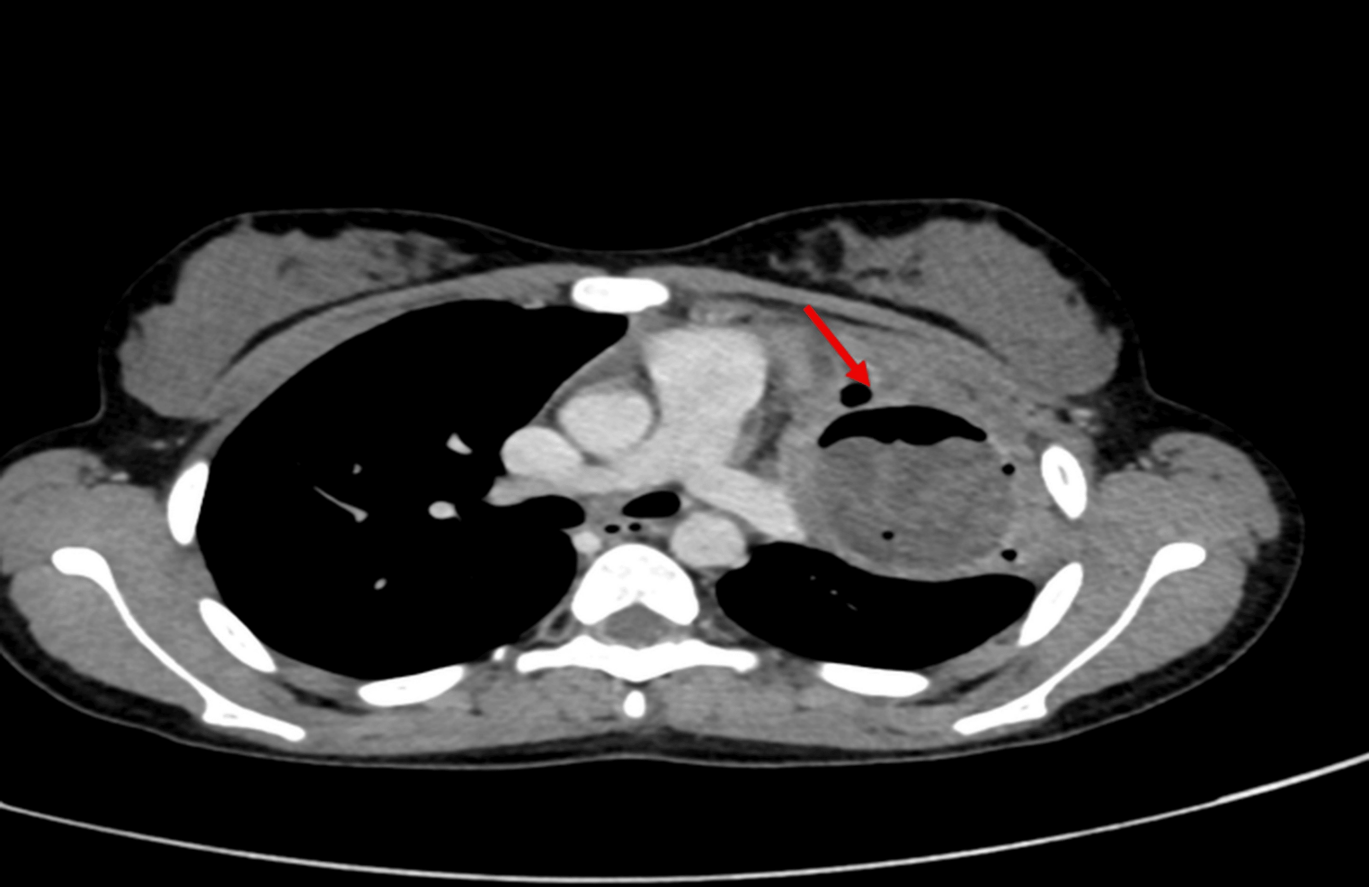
Contrast-enhanced computed tomography (CECT) of the thorax shows a crescent-shaped air pocket in the non-dependent portion of the lesion. There is a well-defined, thick-walled cystic lesion measuring approximately 5.5 x 5.6 x 5.9 cm (transverse x anteroposterior x craniocaudal) in the apical segment of the left upper lobe.
